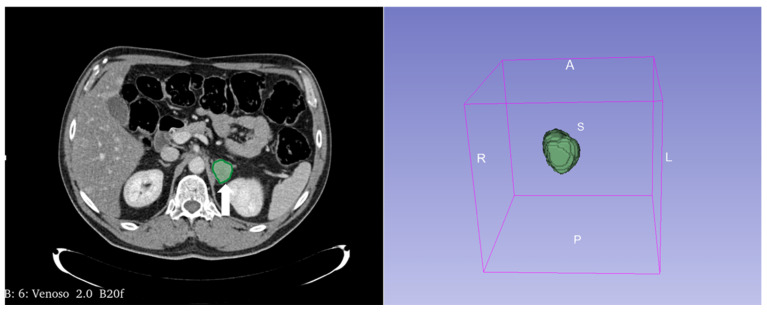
Segmentation of the metastatic lesion (arrow) of the left adrenal gland (same patient of Figure 4) on venous phase CT, using 3D-Slicer Software, version 4.11.
